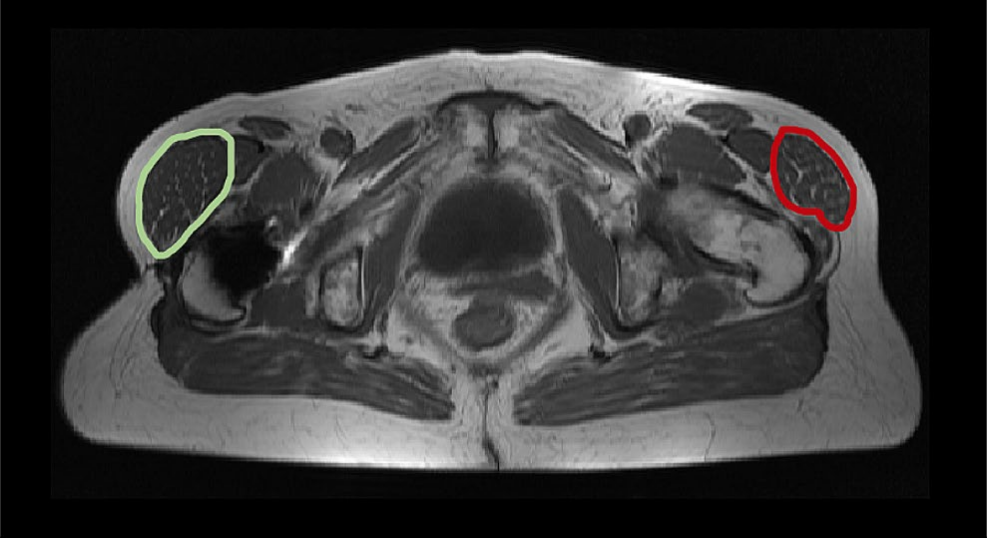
Example of postoperative measurements compared to the unaffected side. Postoperative MRI of the pelvis (T1, transversal plane) of a 56-year-old patient with a chronic gluteal deficiency of the right abductor mechanism after total hip arthroplasty treated with a gluteus maximus transfer. The MRI images 11 months after surgery presenting a compensatory growth of the right musculus tensor facia lata (green) compared to the opposite and unaffected left side (red)
